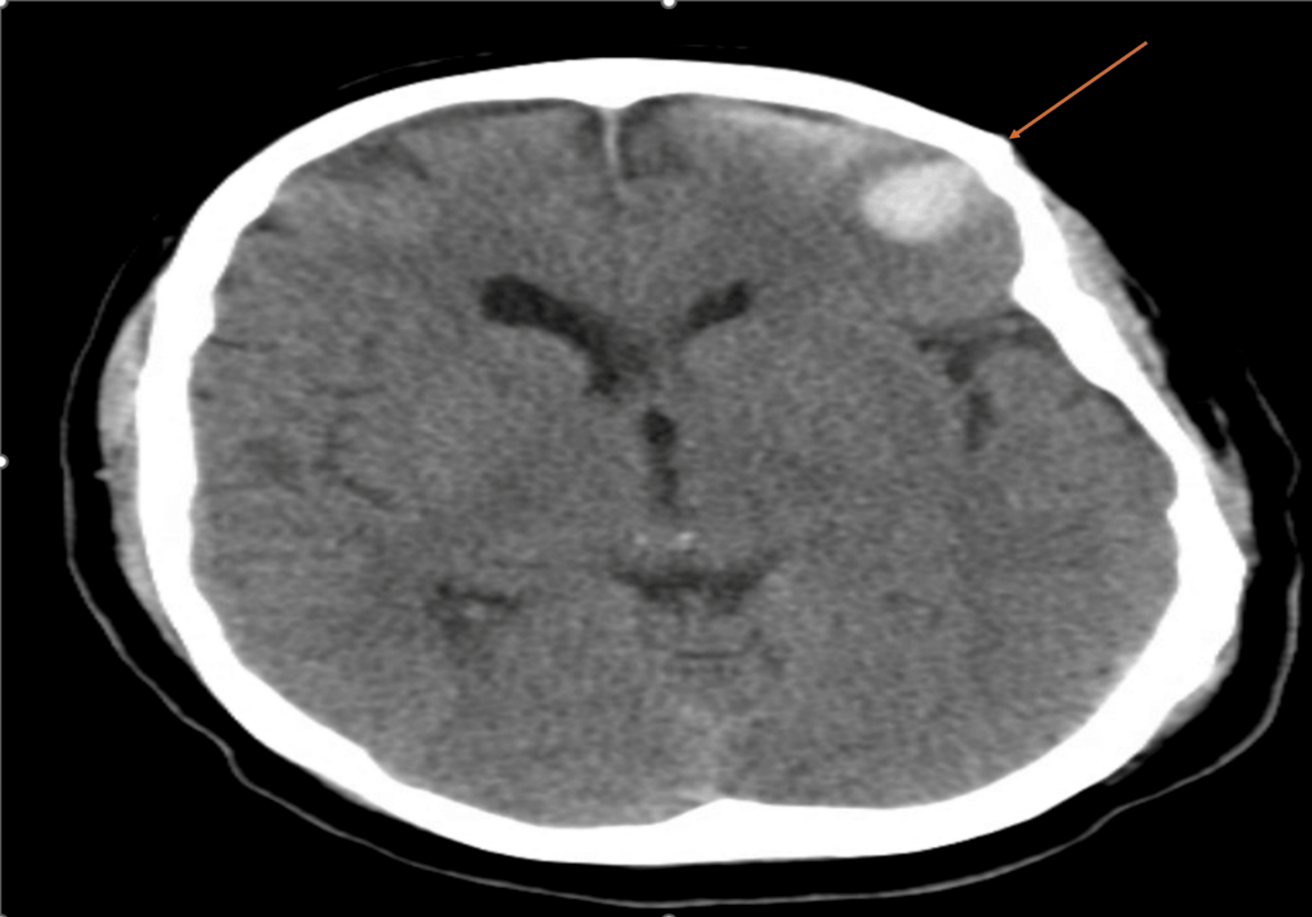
Follow-up CT demonstrating hemorrhage stability (arrow) Arrow demonstrates interval stability and early improvement in traumatic intracranial hemorrhage on follow-up imaging.
